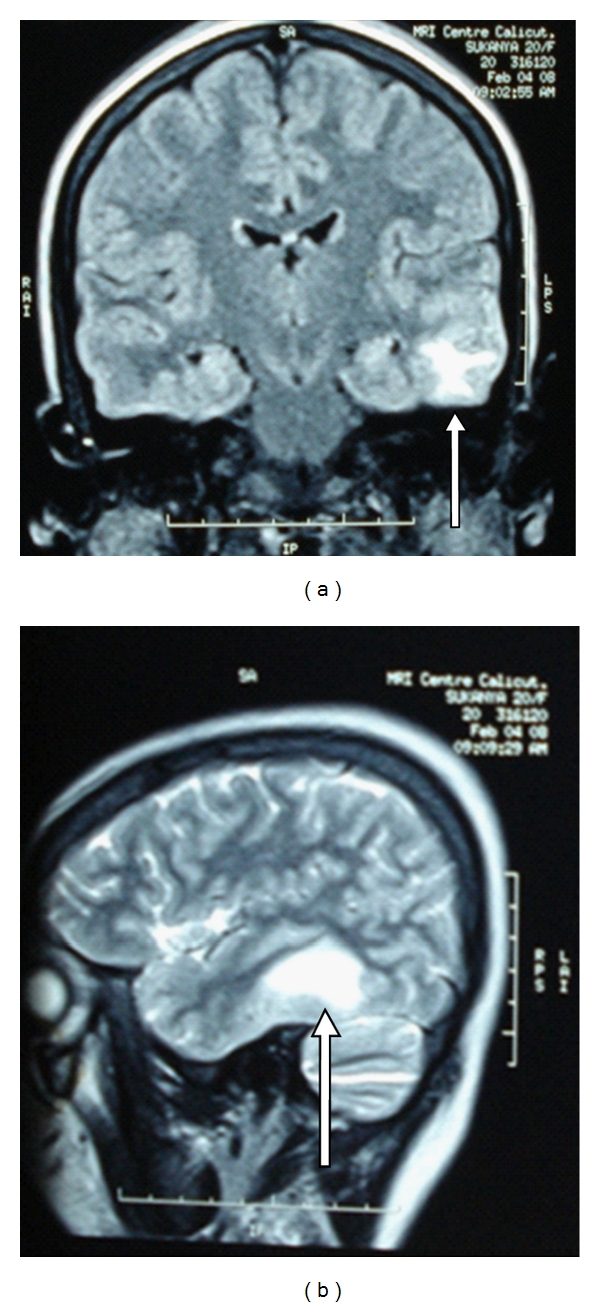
Hyperdense lesion in T2 weighted MRI scan suggesting glioma.
